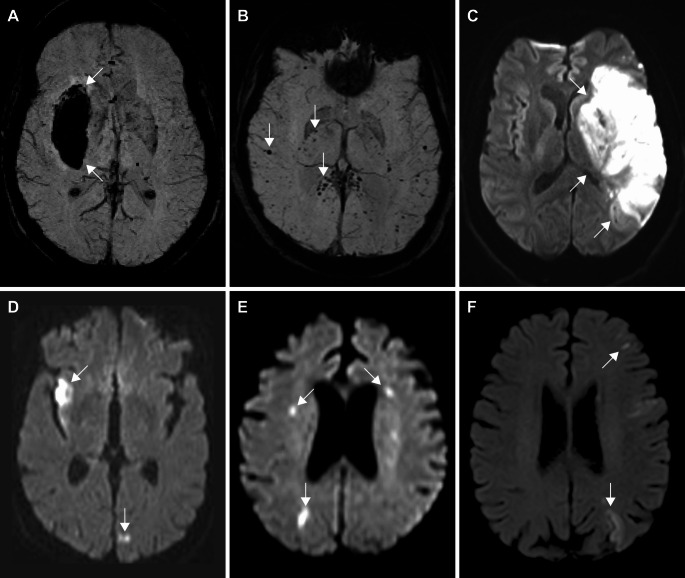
Hemorrhagic and ischemic patterns: SWI (a, b), axial diffusion (c-f) weighted MR images. A: intraparenchymal hematoma (arrowhead; pattern D1), b: cerebral microbleeds with involvement of internal capsules, corpus callosum splenium and sub-cortical white matter (arrowheads; pattern D2), c: left middle cerebral artery infarct: single territory large vessel infarct (arrowhead; pattern E1), d: multiple territories large vessel infarcts (arrowheads; pattern E2), e: multiple acute small vessel infarcts (arrowheads; pattern E4), f: border zone infarcts (arrowheads; pattern E5)
